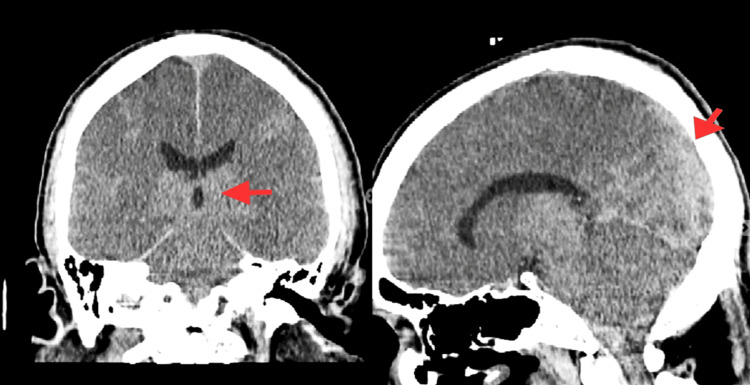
Initial non-contrast CT brain at admission showing diffuse loss of gray-white differentiation and slit-like ventricles, concerning for early cerebral edema Red arrows indicate areas of cerebral swelling.
